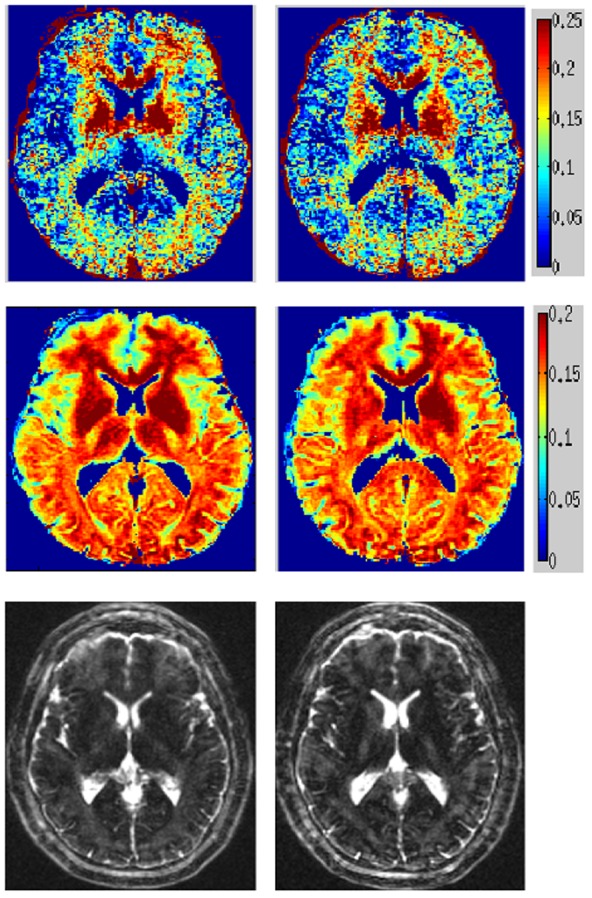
MWF maps of another healthy 3D T2prep adiabatic spiral brain scan. Another healthy brain 3D T2prep adiabatic spiral example, with MWF (top) and T2-weighted image (bottom) of two adjacent axial slices.
